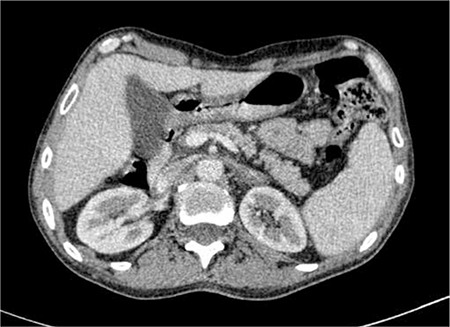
CT scan at the end of treatment showed a complete response.
